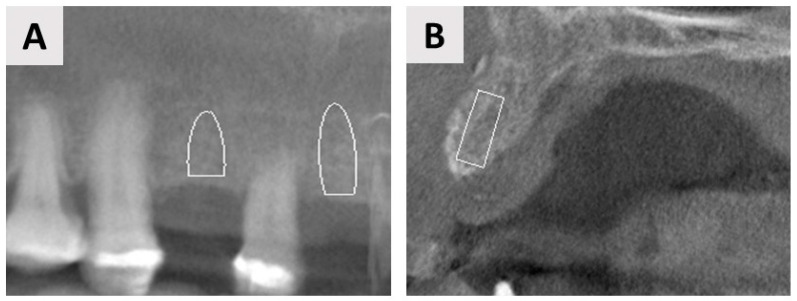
(A) A panoramic CBCT section shows bony healing in the vertical dimension. (B) Coronal CBCT section shows good bone regeneration in the vestibulo-oral dimension. White lines shows planned implantation site.
